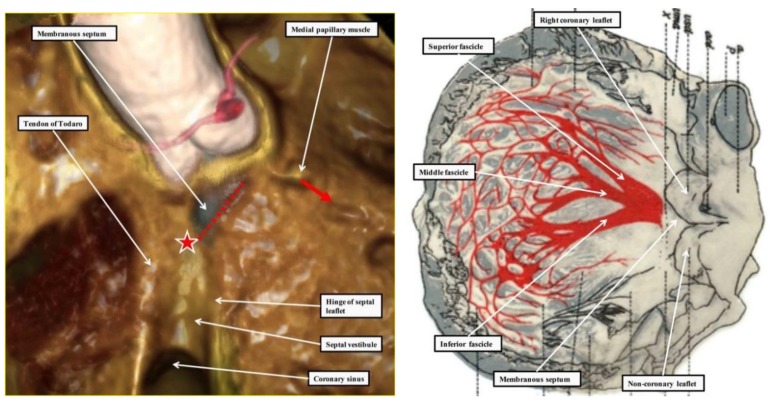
The left-hand image, prepared from a computed tomographic dataset obtained from an individual undergoing investigation for coronary arterial disease, shows how the location of the atrioventricular conduction axis can be predicted based on knowledge of the landmarks of the right atrial and right ventricular septal surfaces. The star shows the location of the atrioventricular node at the apex of the triangle of Koch. The red dotted line shows the location of the non-branching and branching components of the axis, which are carried on the crest of the muscular ventricular septum. The red arrow shows the site of emergence of the right bundle branch, marked by the medial papillary muscle of the tricuspid valve. The right-hand image is from the original monograph of Tawara [1]. It has been scanned and rotated through 90 degrees relative to the original, placing the image in more attitudinally appropriate orientation. The reconstructions made by Tawara show the origin and distribution of the left bundle branch within the left ventricle.
